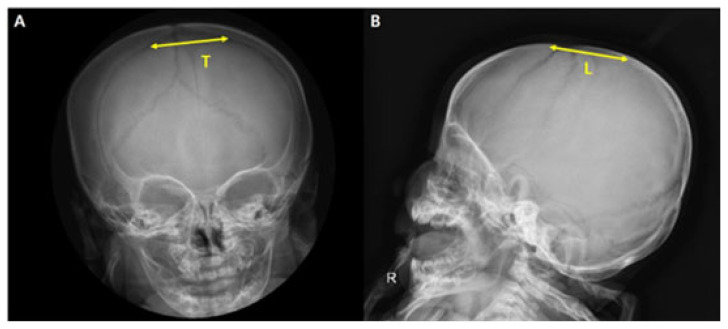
Radiographs of skull in anteroposterior (A) and lateral (B) views are shown. Abbreviation: T = Transverse diameter, L = Longitudinal diameter. Size of anterior fontanelle (SAF) = T + L/2.
